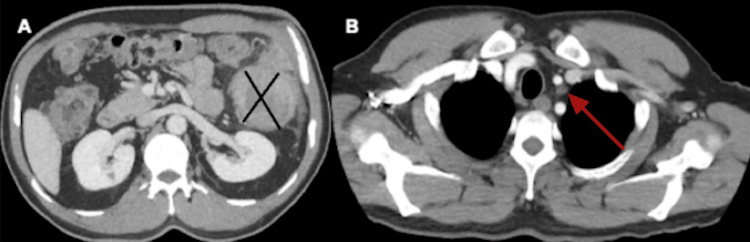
Cross-sectional imaging findings (A) Contrast-enhanced CT of the abdomen demonstrating a large, partially obstructive mass at the splenic flexure with extramural extension. (B) CT of the chest showing mild mediastinal lymphadenopathy, without radiographic evidence of distant metastatic disease. CT: computed tomography
